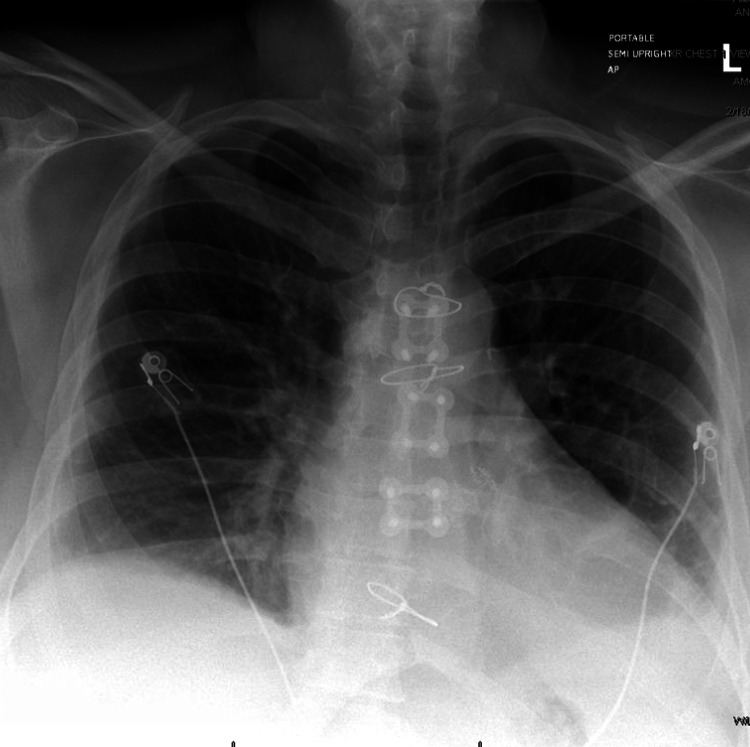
Chest X-ray (portable semi-upright, AP view) The cardiomediastinal silhouette remains unchanged in size and morphology. A prosthetic aortic valve is again noted. Small bilateral pleural effusions are present, with no evidence of pneumothorax. The left pleural effusion has mildly decreased in size compared with the most recent prior exam.
